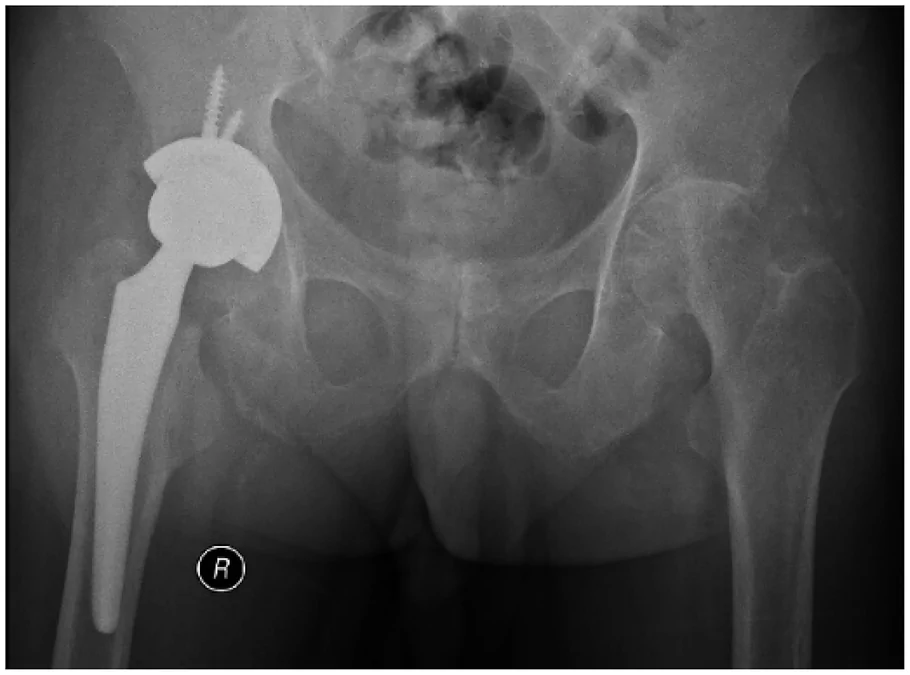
Postoperative anteroposterior radiograph of the hip (Tianjin zhengtian medical equipment Co., Ltd.).
